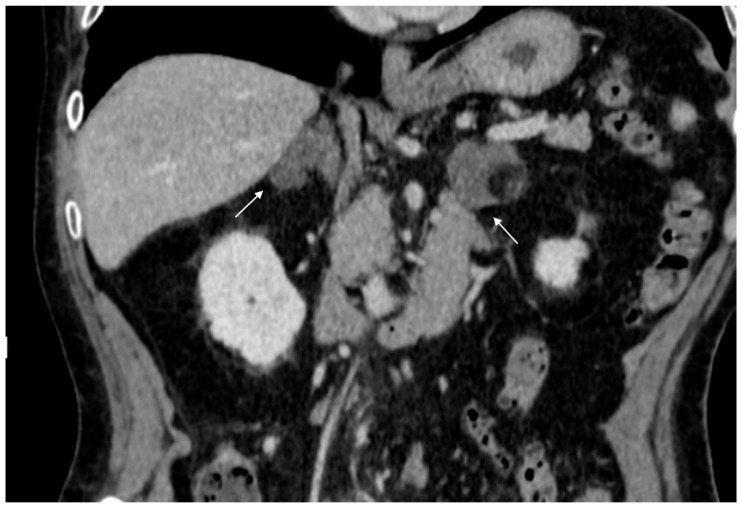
74-year-old male patient. Following an MRI of the digestive tract incidental finding of bilateral adrenal secondary hyperplasia. Both enlarged limbs adrenal glands > 10 mm thick with multiple nodularity hypodense on non-contrast CT (arrows).
